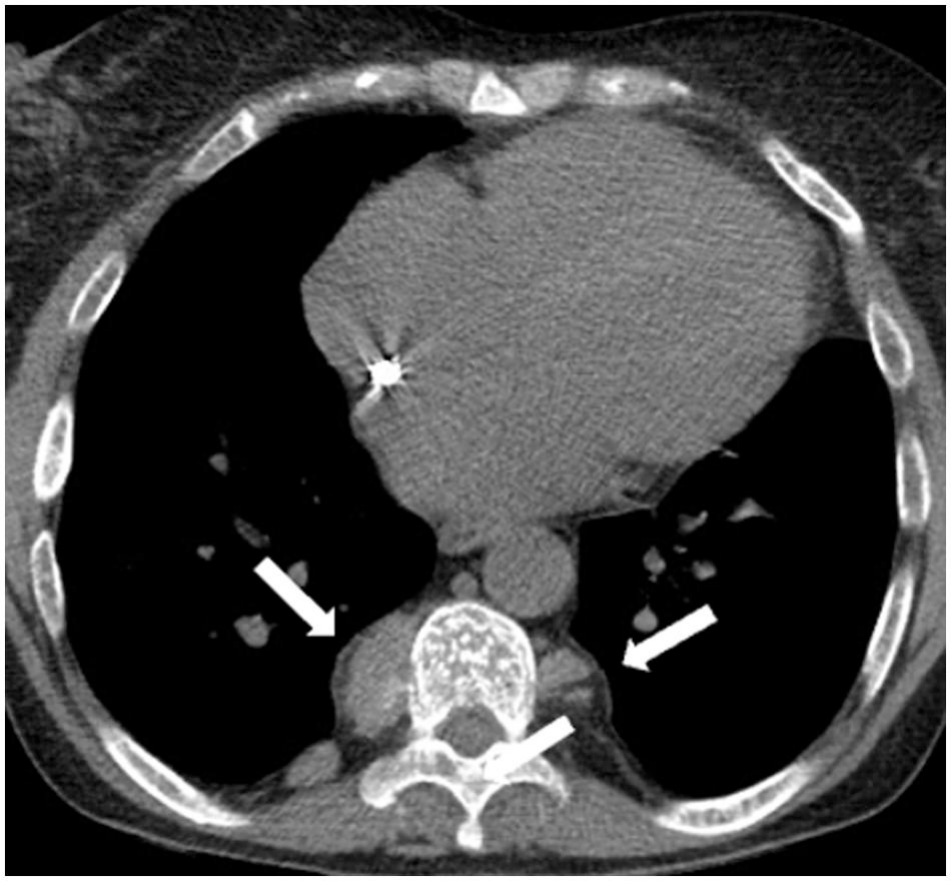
Extramedullary haematopoiesis. Non-contrast axial CT image shows bilateral spinal soft-tissue (arrows) in a patient with beta-thalassemia.
